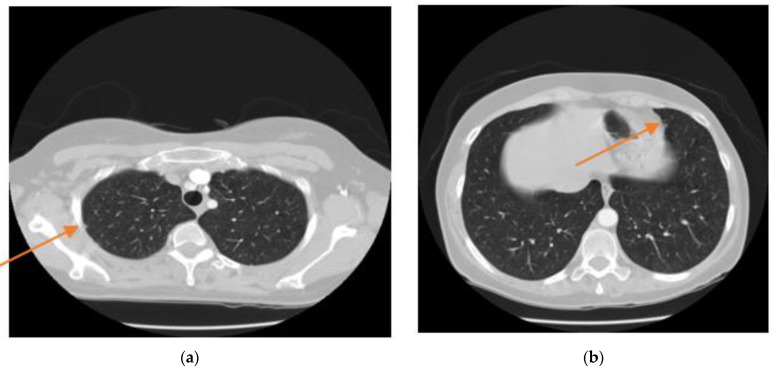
Two examples of nodules attached to the lung wall in CT-scan images. (a). represents one nodule attaching to the outer wall of the lung, (b). represents one nodule attaching to the outer wall of the lung (orange arrows).
